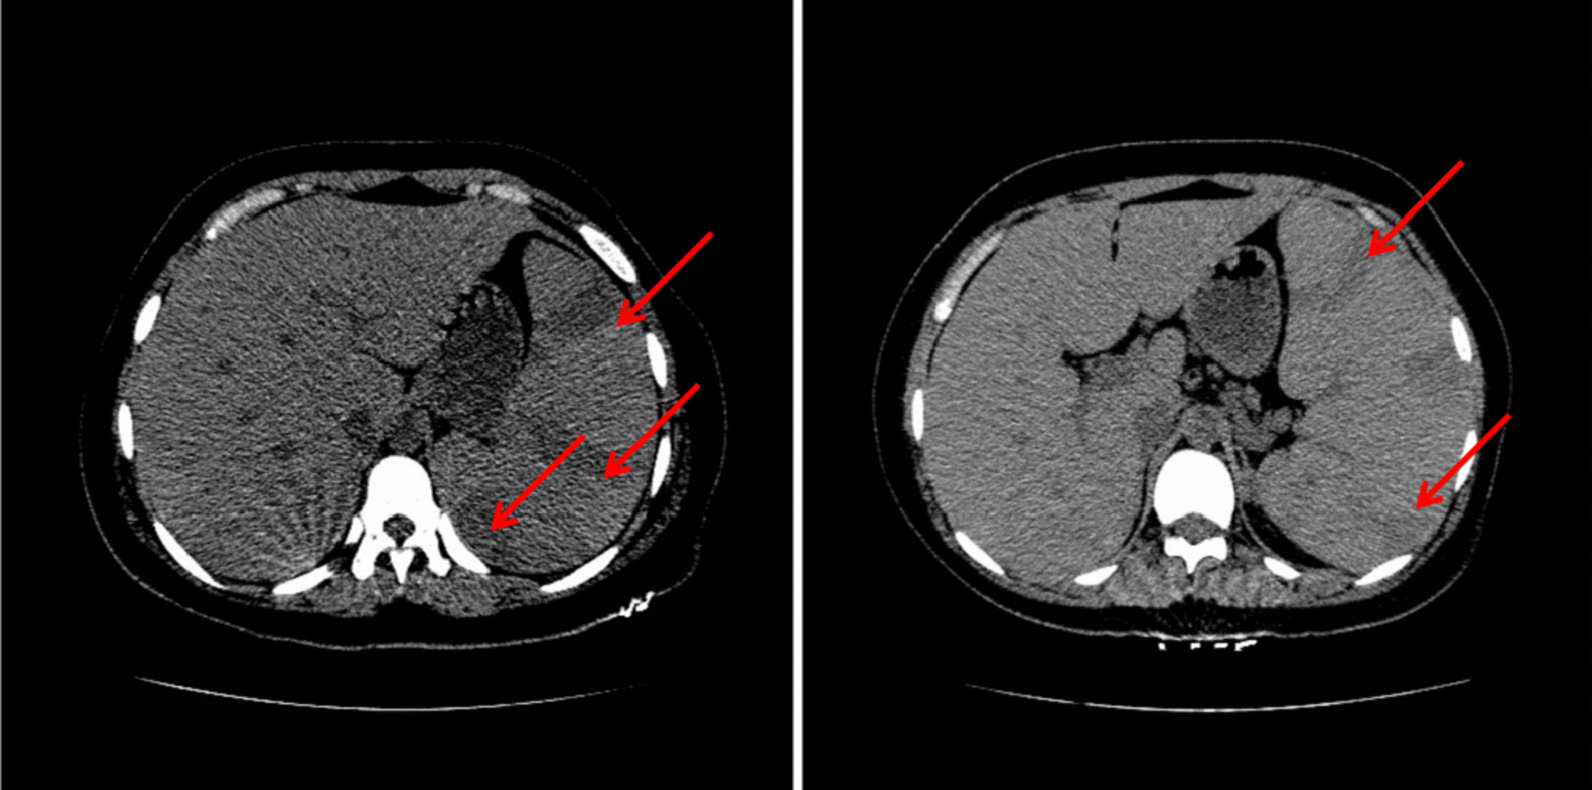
The CT at abdomen showing that the spleen was enlarged with multiple wedge-shaped low-density foci, suggesting the presence of splenic infarction.The arrows point to the splenic infarction
